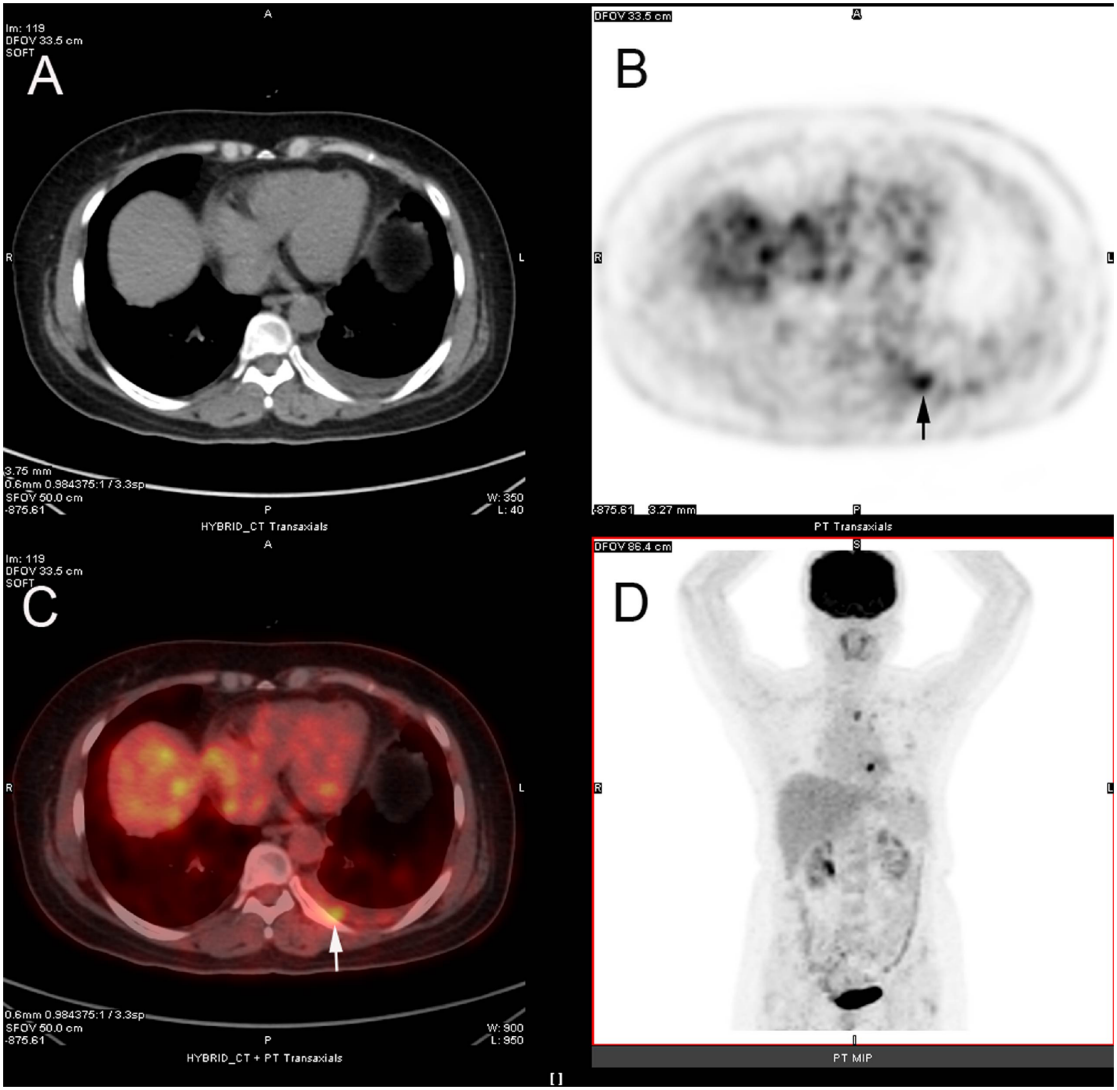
^18^F-FDG PET/CT integrated imaging of 54-year old woman with left lung cancer and malignant pleural effusion. Axial CT (A) shows effusion in left pleural cavity, and axial ^18^F-FDG PET (B, arrow) and axial fused ^18^F-FDG PET/CT (C, arrow) display nodular ^18^F-FDG uptake (SUVmax of 3.0) in left-posterior pleural region. Pathology from thoracentesis confirmed malignant pleural effusion caused by metastatic adenocarcinoma.
